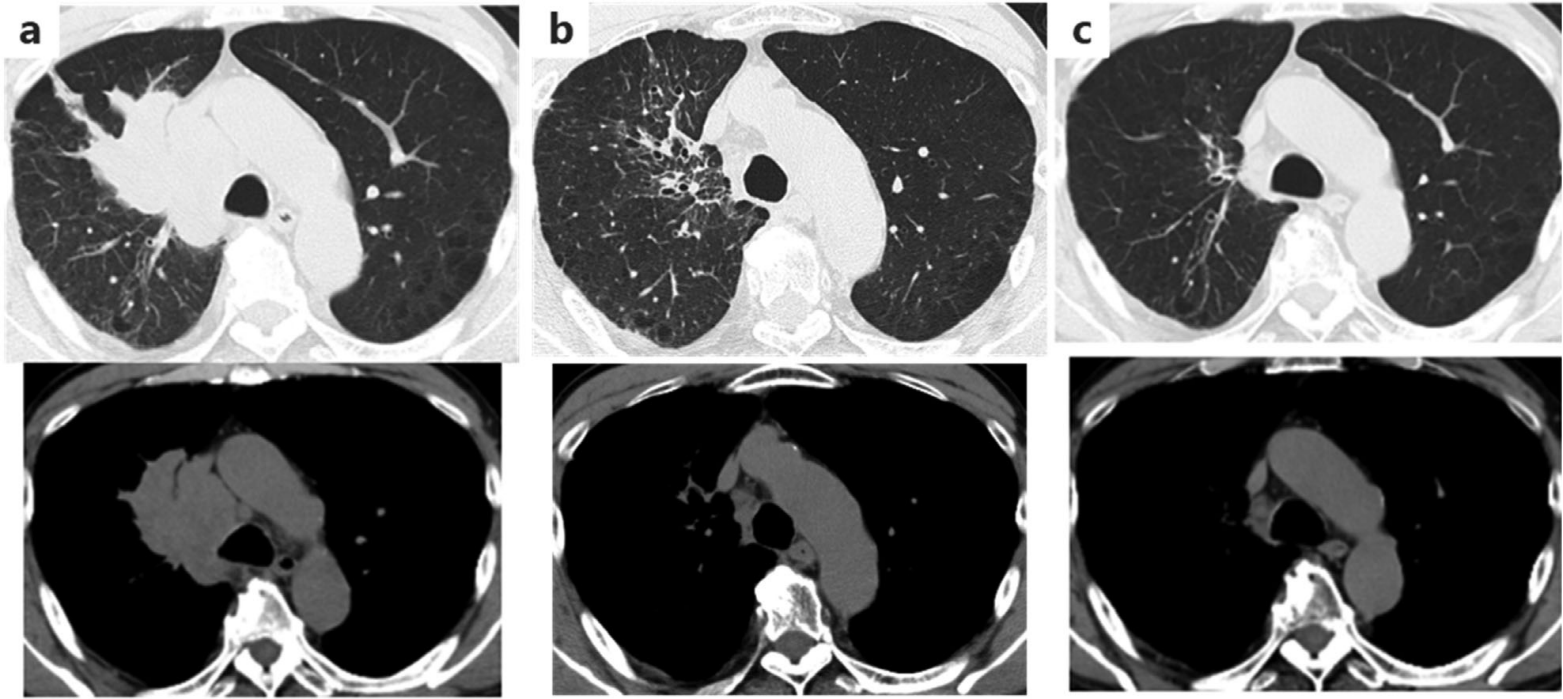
Chest computed tomography (CT) images (a) at diagnosis; (b) before administration of durvalumab; and (c) at the onset of immune‐related myositis after the third course of durvalumab treatment when the size of the lung mass had decreased dramatically.
